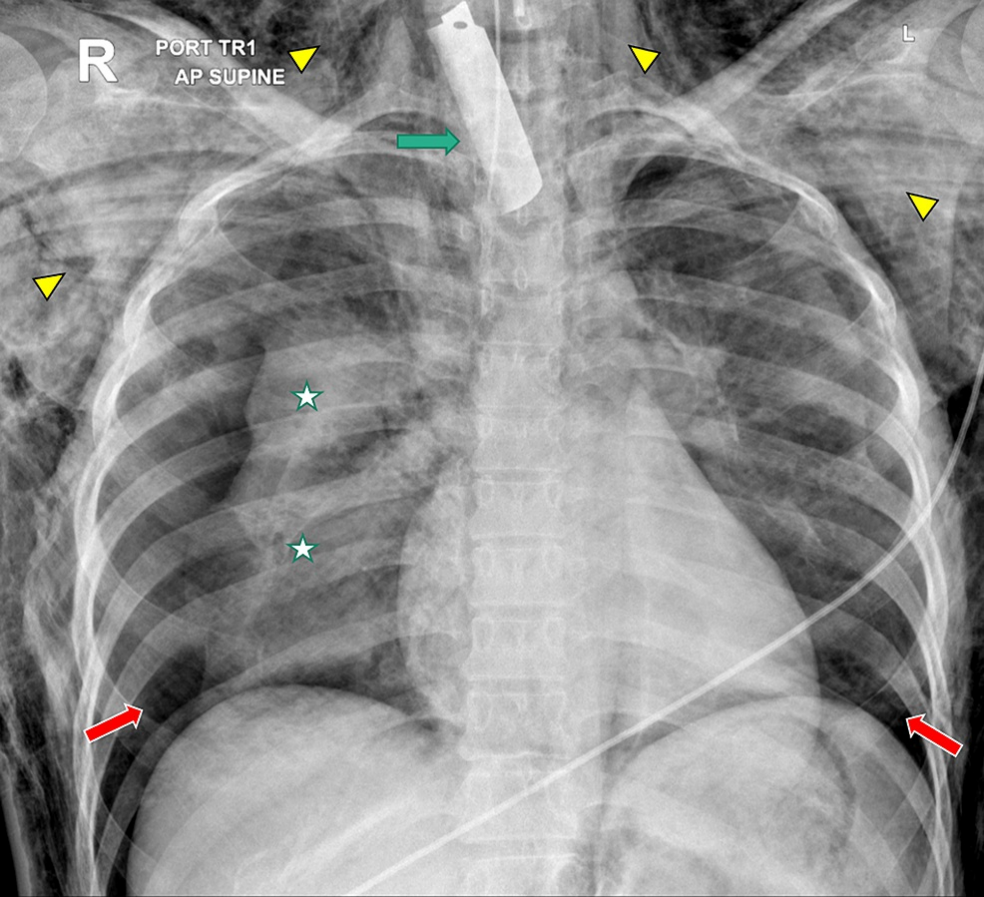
Chest X-ray showing a larger rectangular radiopacity seen projecting over the root of the neck extending to the thoracic inlet in the midline concerning for a foreign body (green arrow), bilateral pneumothorax right larger than the left (red arrows ) with atelectasis of the right lung (star), and extensive surgical emphysema in the chest wall (yellow arrowhead).
